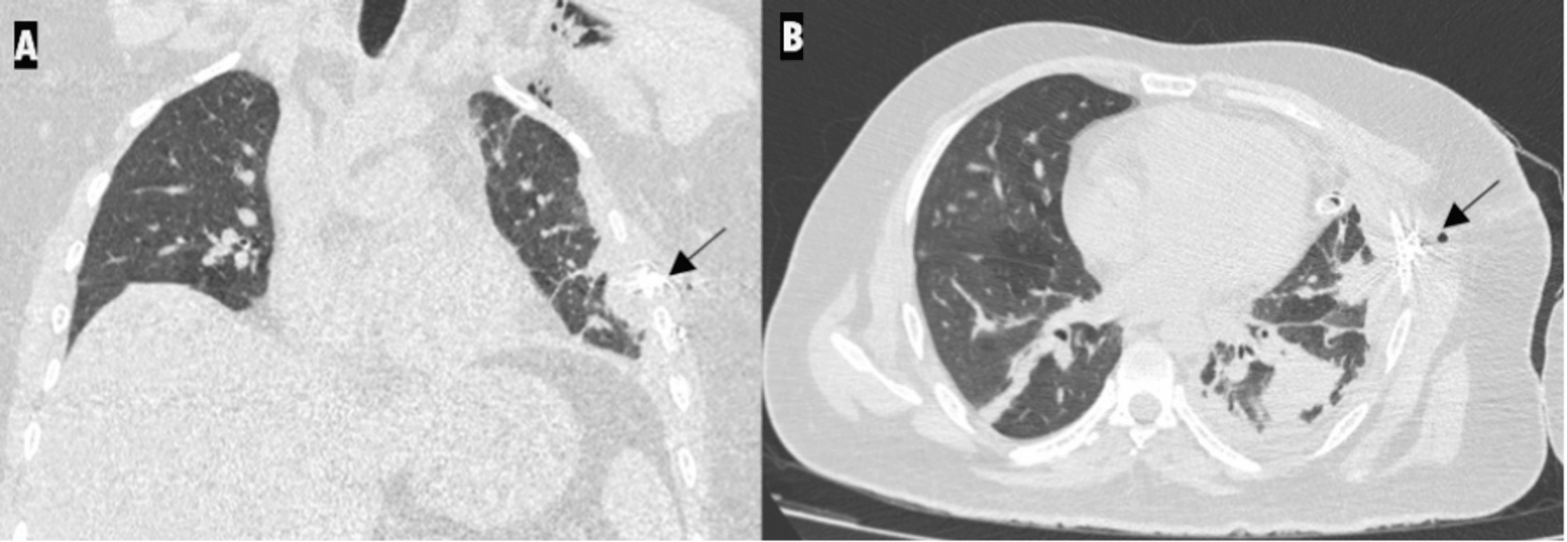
Coronal (A) and axial (B) chest CT images (post-operative day 4) show pleural collection with resolving contusions in the lingula and left lower lobe. Post-operative changes are seen in the left fourth and fifth ribs (black arrow). Residual subcutaneous emphysema is also noted. The ICD is seen "end on". ICD: implantable cardioverter defibrillator
